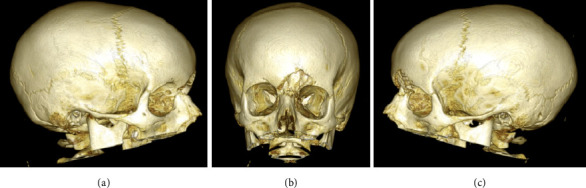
3D maxillofacial CT scan reconstruction allows for a better visualization of the extent and location of the frontal bone resorption.
